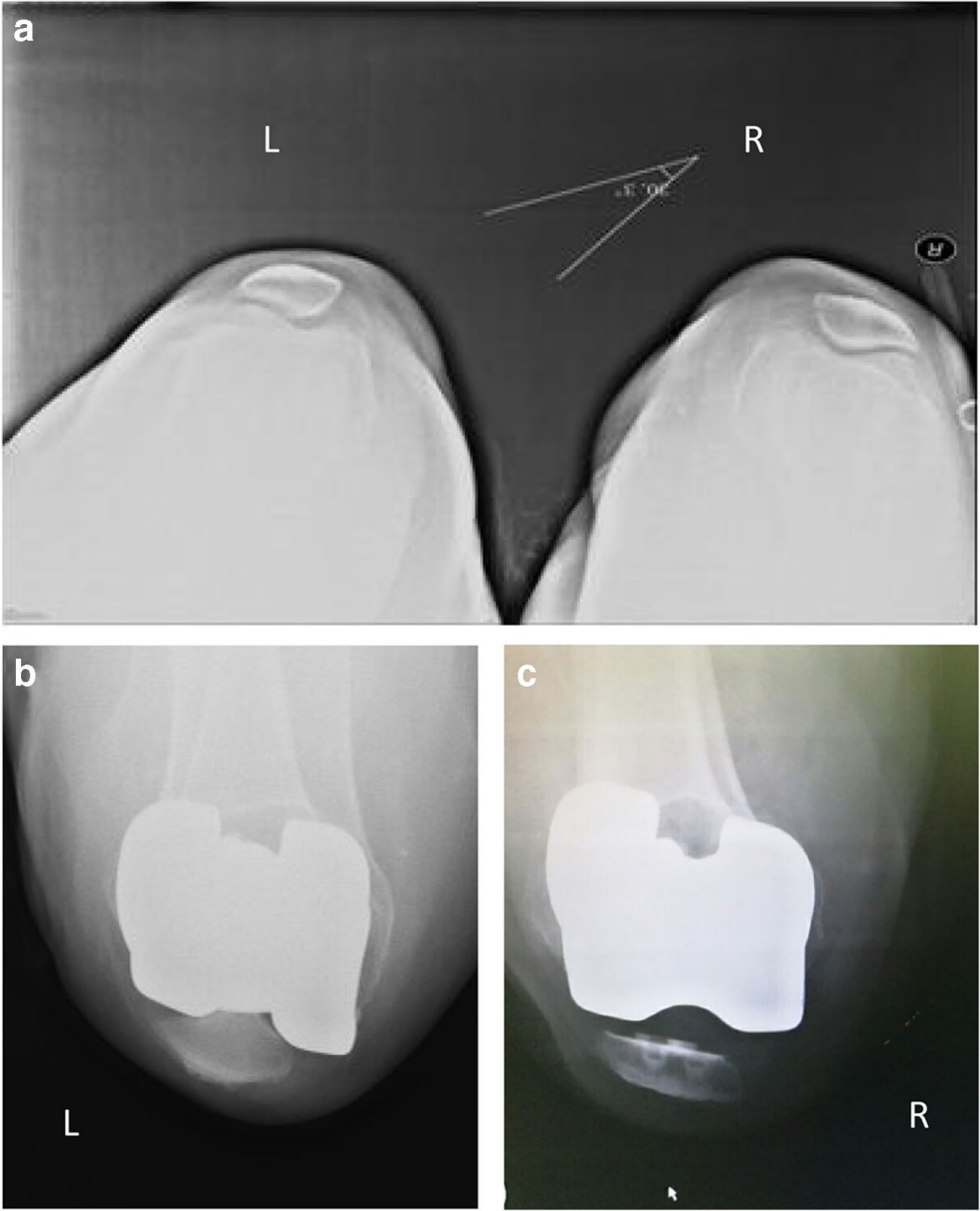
Pre-operative (3 days) and post-operative (1 month) X-ray examinations (axial patella view) of a 68-year-old woman with bilateral osteoarthritis. Left: non-resurfaced patella. Right: resurfaced patella. One-month after the operation, the patient’s pain was significantly relieved, the activities were recovered well, and the post-operative joint flexion activity reached 110°. a Axial position 3 days before operation. b Left knee joint in the axial position 1 month after operation. c Right knee joint in axial position 1 month after operation
